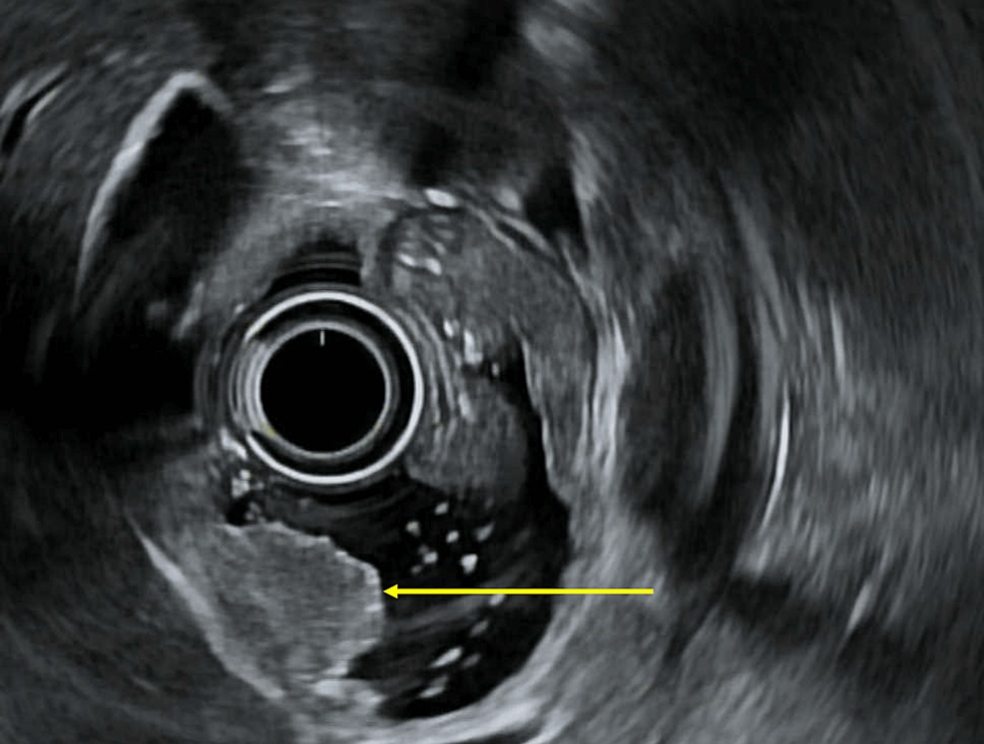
Endoscopic ultrasound demonstrating a 15 mm lesion in the deep mucosa.
